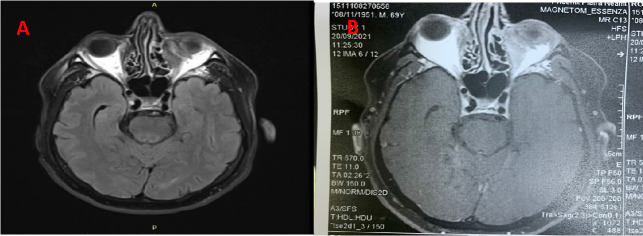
MRI of the orbital inflammatory pseudotumor - before and after treatment (A. 41/ 20/ 15 mm; B- 31/ 10/ 12 mm)
